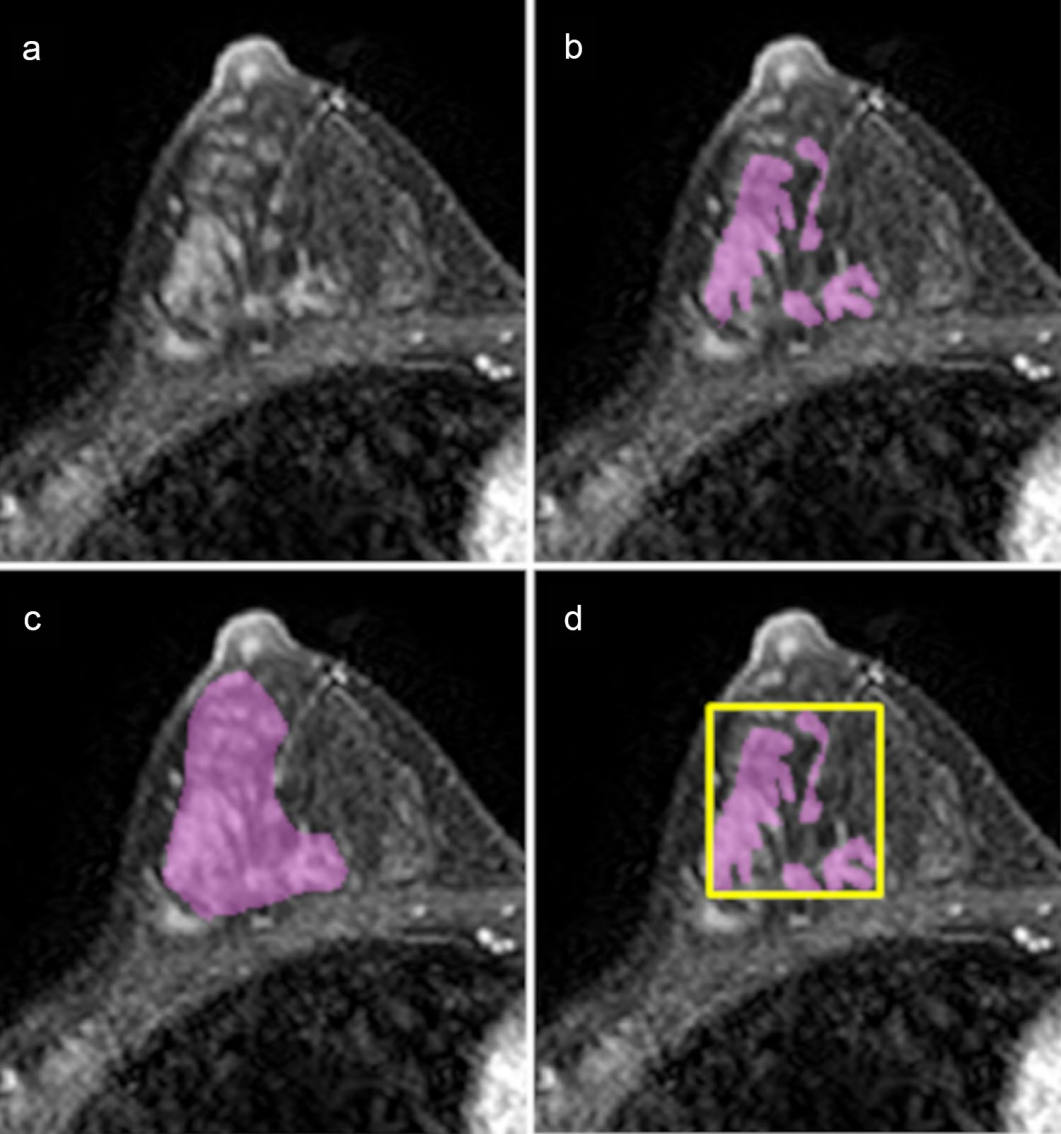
A 49-year-old patient with ductal carcinoma in situ. a Axial early-phase dynamic contrast-enhanced MRI demonstrates non-mass enhancement in the right breast. Reader 1 (highly experienced radiologist) assessed the lesion with segmental distribution and clustered ring internal enhancement, and finally decided on BI-RADS category 5. Two patterns of the ROI were set by one radiologist: b ROI-1, precise segmentation, which contains only the enhancing lesion, and c ROI-2, rough segmentation that covers the whole enhancing region, including the intervenient non-enhancing area. The input bounding boxes for ResNet50 were generated with the entire lesion d for all slices
